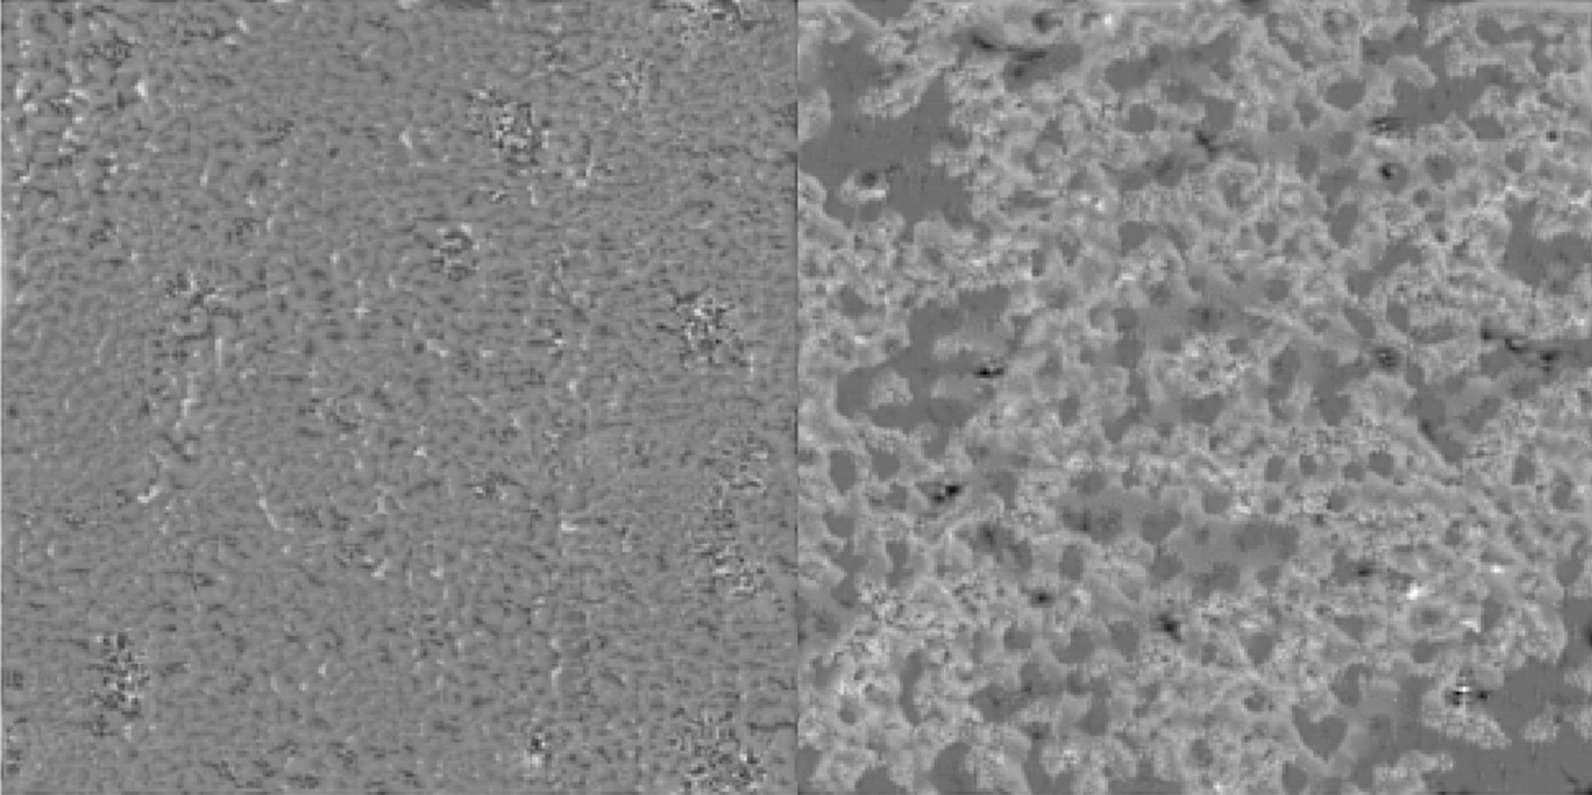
SegNet binary segmentor Deep Dream image Deep dream image laying out key features the network is using to segment the CT scans. infected tissue (right), non-infected (left)
